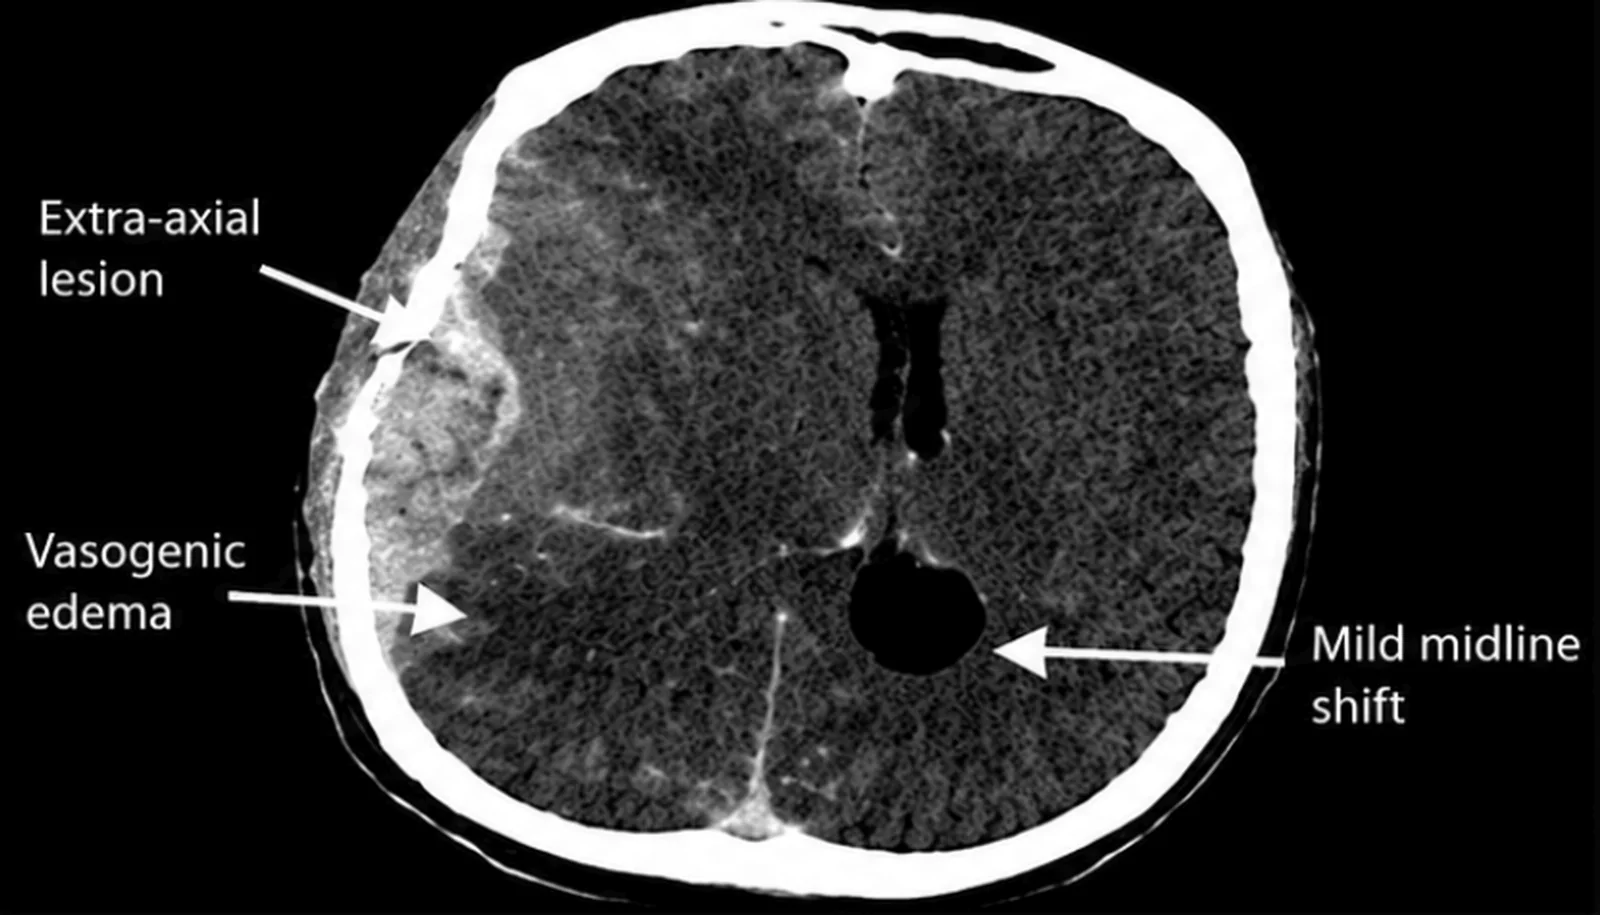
Preoperative contrast-enhanced CT scan. Axial contrast-enhanced computed tomography (CT) demonstrating a right frontotemporoparietal extra-axial lesion (white arrow) associated with extensive vasogenic edema (arrowhead) and mild leftward midline shift, initially suggesting a meningioma en plaque.
